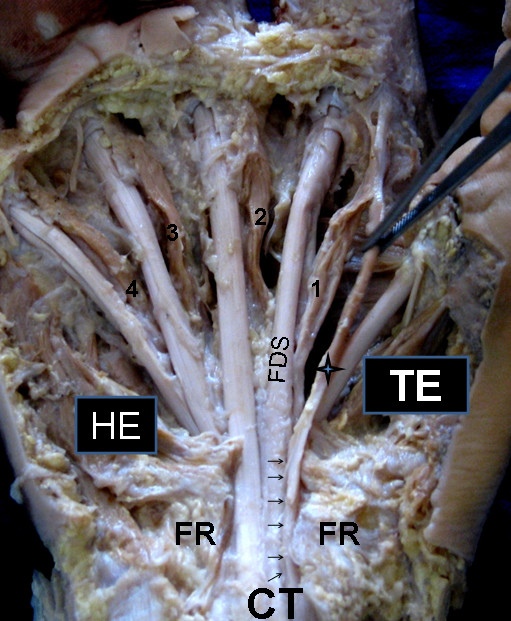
Right side palmar surface of the hand. 1, first lumbrical; 2, second lumbrical; 3, third lumbrical; 4, fourth lumbrical; CT, carpal tunnel; FDS, flexor digitorum superficialis tendon to the index finger; FR, flexor retinaculum; HE, hypothenar eminence; TE, thenar eminence. Note the 4-point star indicates the additional muscle belly of the first lumbrical muscle.
